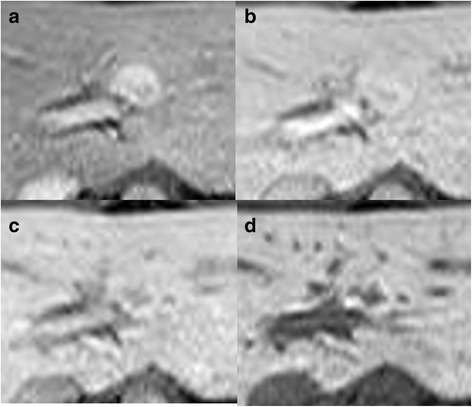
Hemodynamics of liver tumor on Gd-EOB-DPTA-enhanced MRI. Hyper-vascularity is shown on a arterial phase, b portal venous phase, and c delayed phase. d On hepatocyte phase, the hypo-intense lesion is shown with a ring-shaped hyperintense lesion
